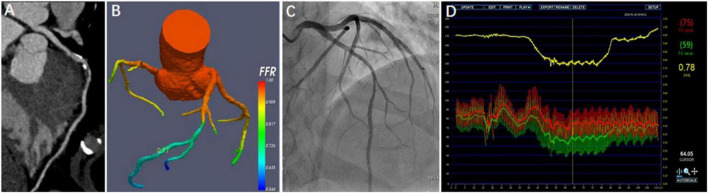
Representative case from the study. A 45-year-old man with atypical angina. CTA showed a 50–70% stenosis in the middle segment of the left anterior descending artery (LAD) (A), and CT-FFR value (0.77) was measured in the distal of target lesion (B). Invasive coronary angiography (ICA) demonstrated the severe stenosis of 70% (C). FFR measured at the corresponding location was 0.78 (D).
